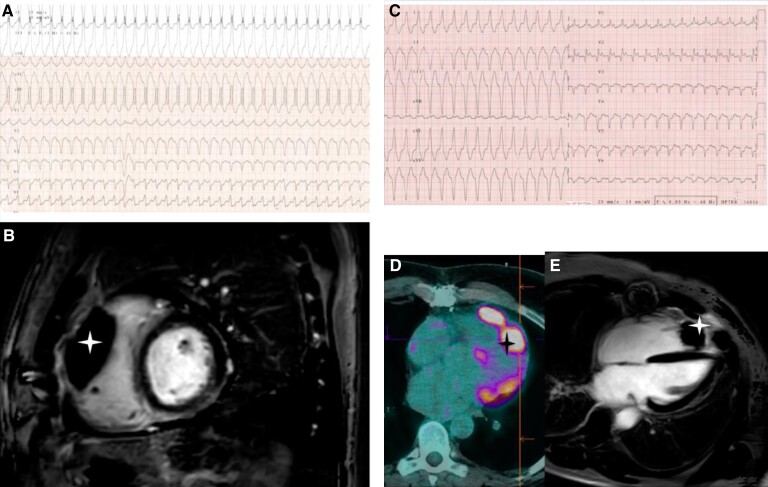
Heart lymphoma imaging and corresponding ventricular tachycardia during electrical storm. (*A*) Ventricular tachycardia from antero-medium node. (*B*) Antero-medium wall node on magnetic resonance imaging (MRI) phase-sensitive T1 inversion recovery (PSIR) sequence small axis plan. (*C*) Ventricular tachycardia from apical node. (*D*) Positron emission tomography 18 fluorodesoxyglucose 4 cavity plan antero-medium and apical nodes. (*E*) Antero-medium and apical nodes on MRI SPIR sequence 4 cavity plan. Stars indicates the VT’s causal nodes.
